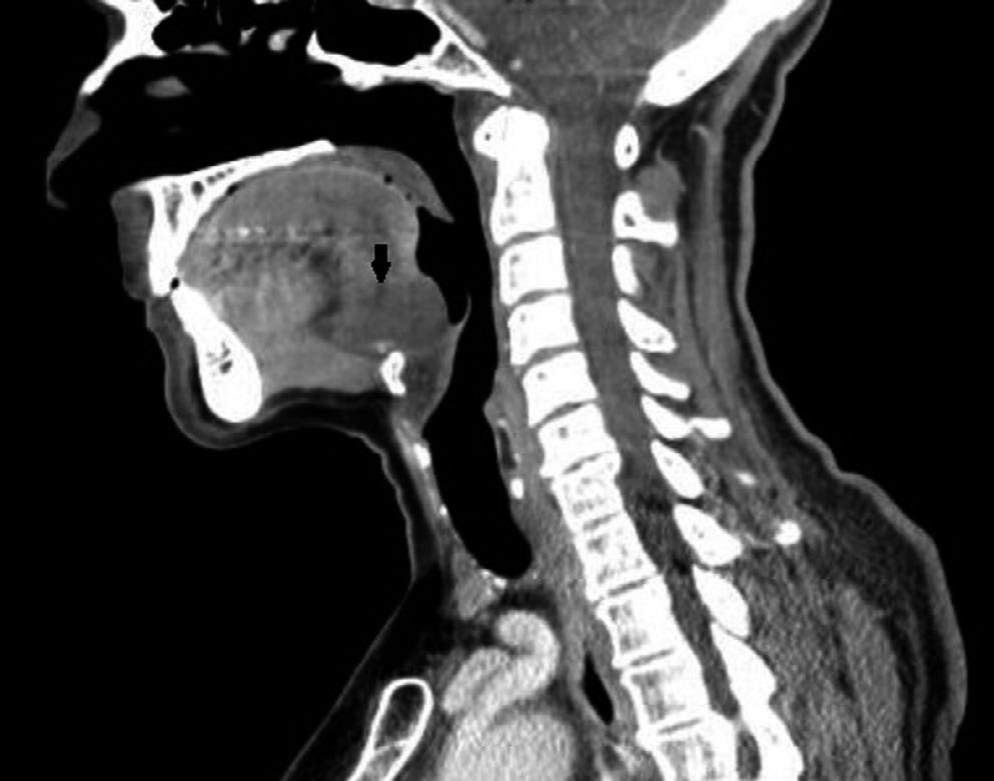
CT scan (sagittal plane)—homogeneous 22 × 18 mm nodular formation in the posterior region of the base of the tongue
